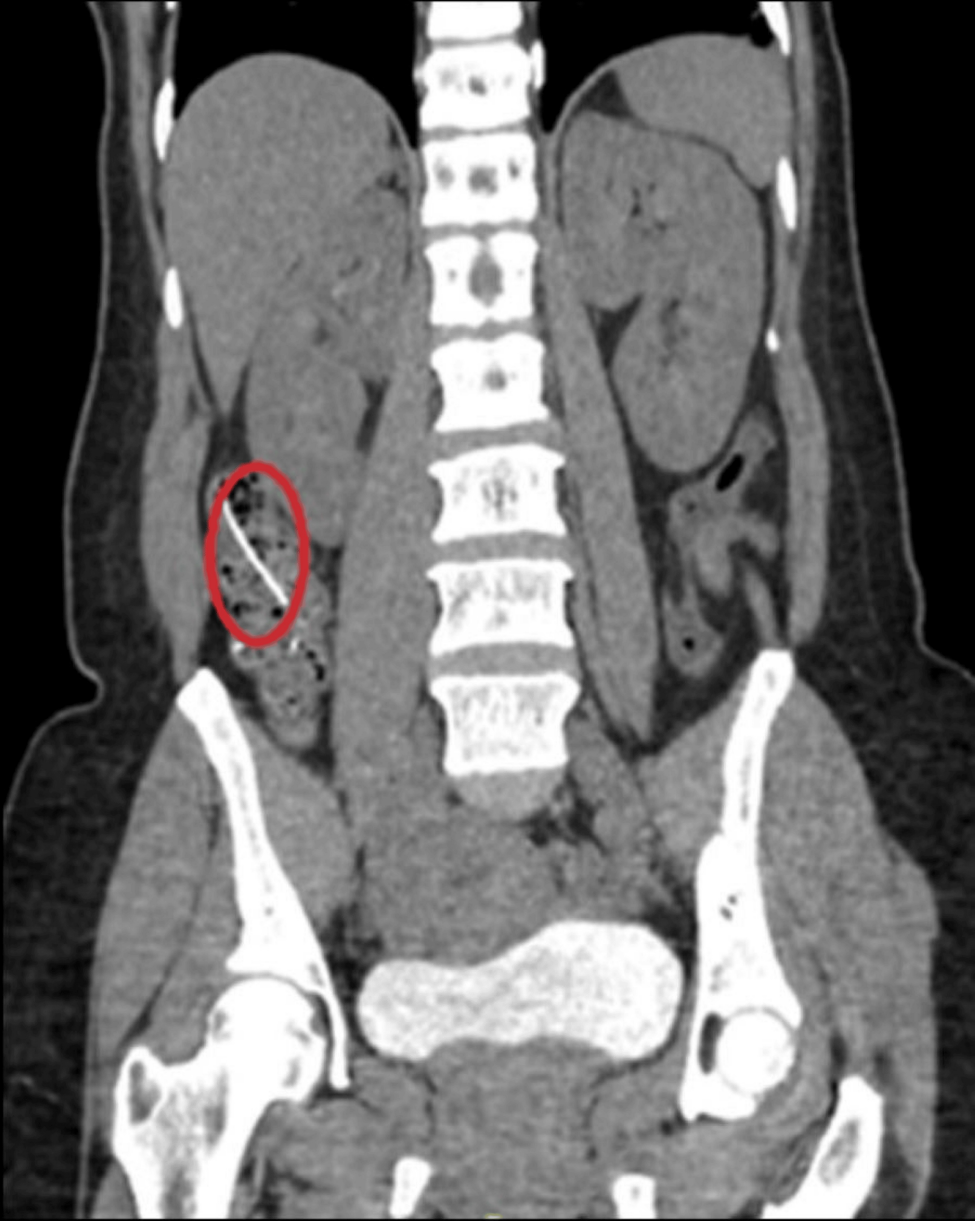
Repeat CT scan on admission day 2 showing a foreign body in the ascending colon
